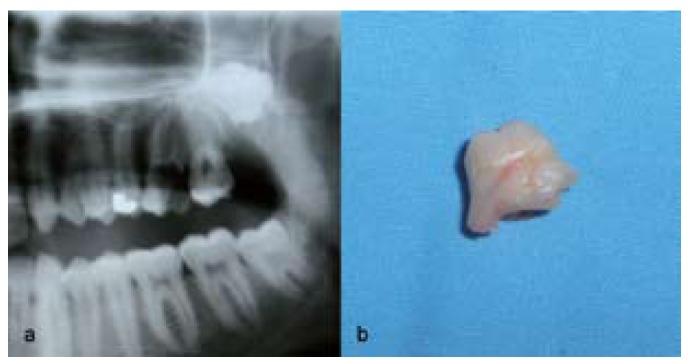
Radiographic appearance of fusion of the third molar and distomolar teeth (a), appearance of fused teeth after operation (b).
